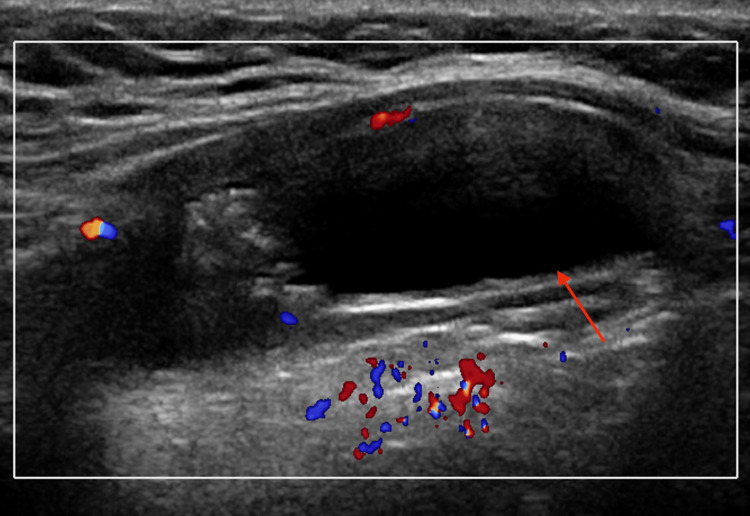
Ultrasound of the neck soft tissues. The ultrasound revealed a complex-appearing cystic structure (red arrow) within the midline of the neck, superior to the thyroid gland and immediately deep to the subcutaneous tissues, measuring 4.1 x 1.4 x 2.1 cm, with no internal vascularity.
